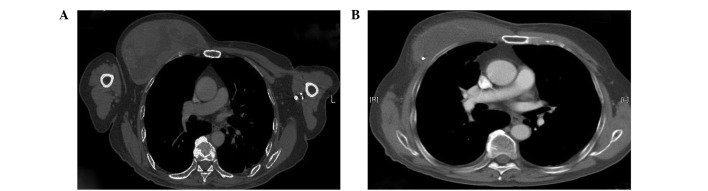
Computed tomography of the thorax showing (A) the tumour prior to CyberKnife radiotherapy and (B) the marked reduction in the tumour size following CyberKnife^®^ radiotherapy.
